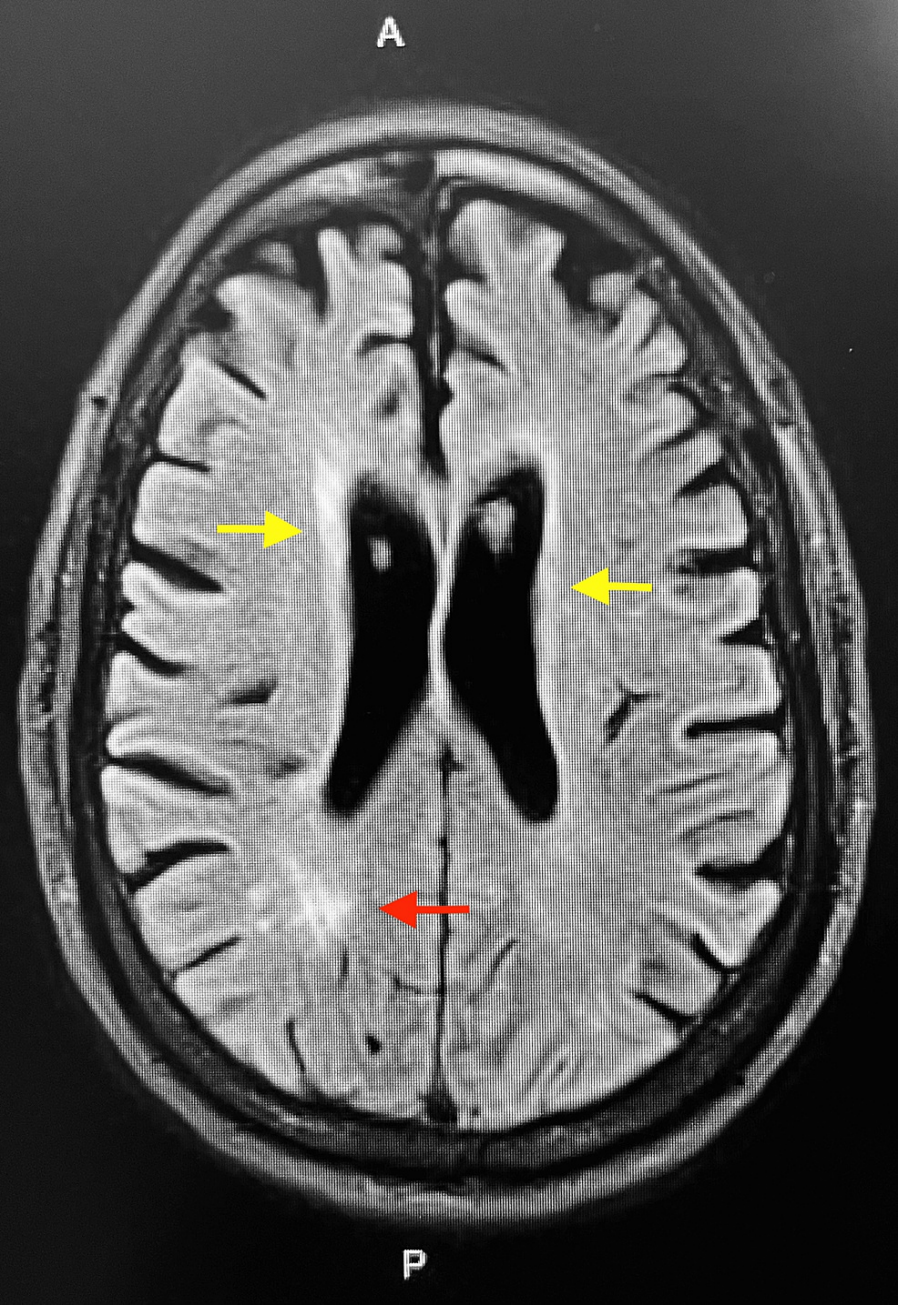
Alternative transverse sequence of MRI Brain showing increased T2 signal within the periventricular (yellow arrows) and subcortical (red arrow) white matter; characteristic of toxic leukoencephalopathy.
